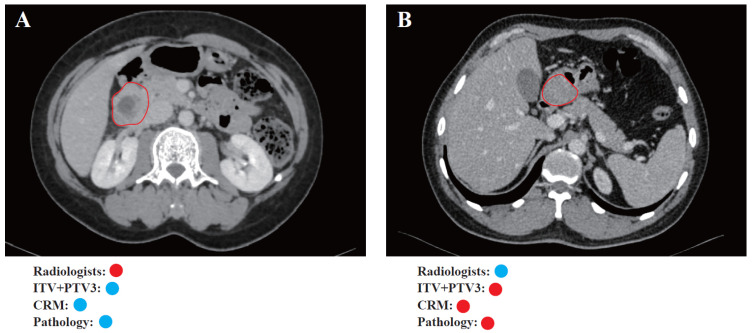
Model performance in GISTs with atypical radiological features. Red circles outline lesions on CT images. Blue dots indicate low-risk GISTs; red dots indicate high-risk GISTs. Case 1 (A): Low-risk GIST (mitotic count=5/50 HPFs) presenting as a 4.5 cm irregular duodenum lesion with necrosis. Rad-score=2473. Nomogram total points=24, high-risk probability=0.3. Case 2 (B): High-risk GIST (mitotic count=10/50 HPFs) presenting as a 4.2 cm regular-shaped intragastric lesion without necrosis. Rad-score=5376. Nomogram total points=32, high-risk probability=0.7. Both cases were misclassified by radiologists but correctly predicted by ITV+PTV3 and CRM.
